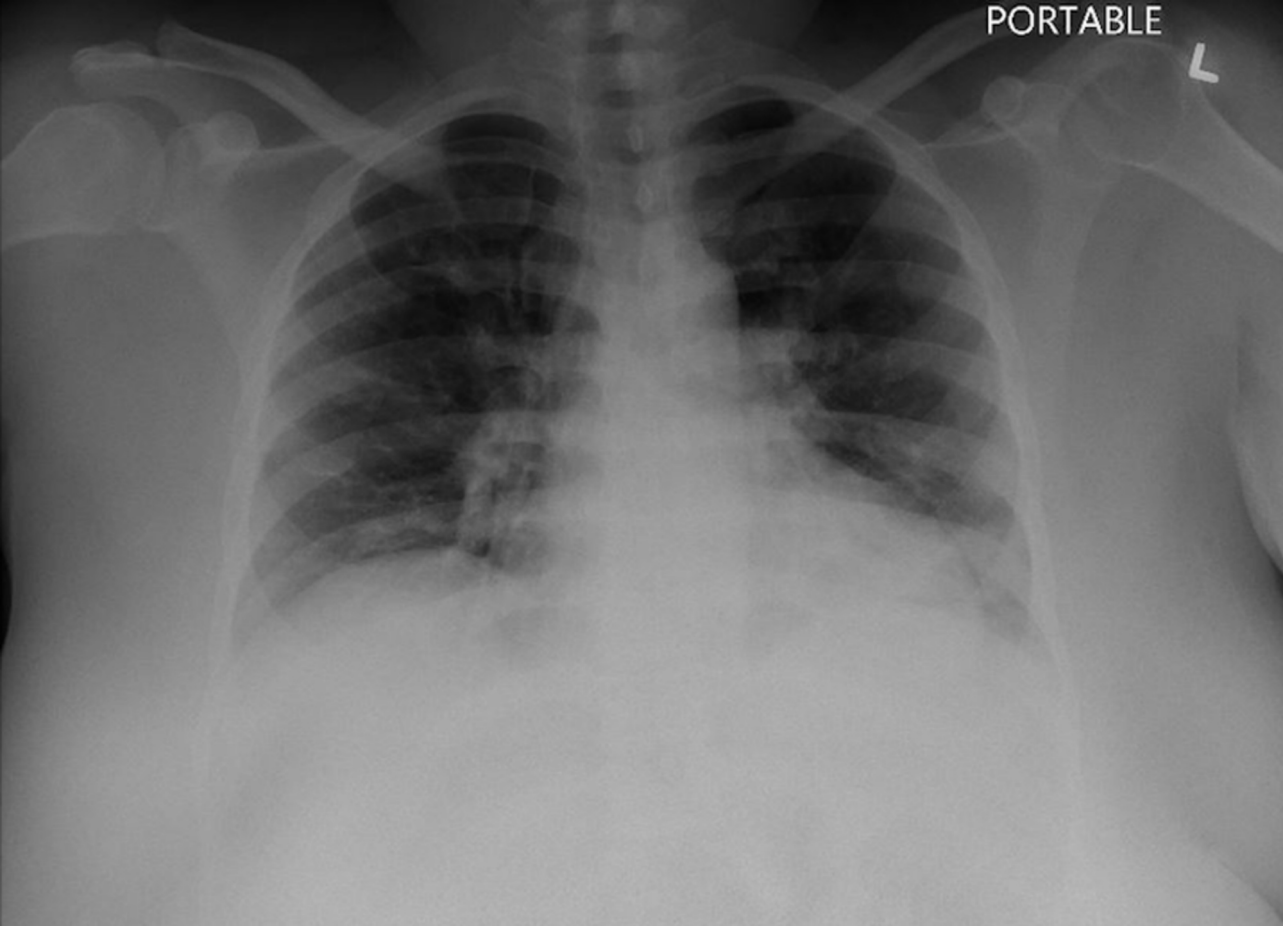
Chest radiography (CXR) from admission showing mild bilateral increased interstitial markings with no opacities or consolidations.
